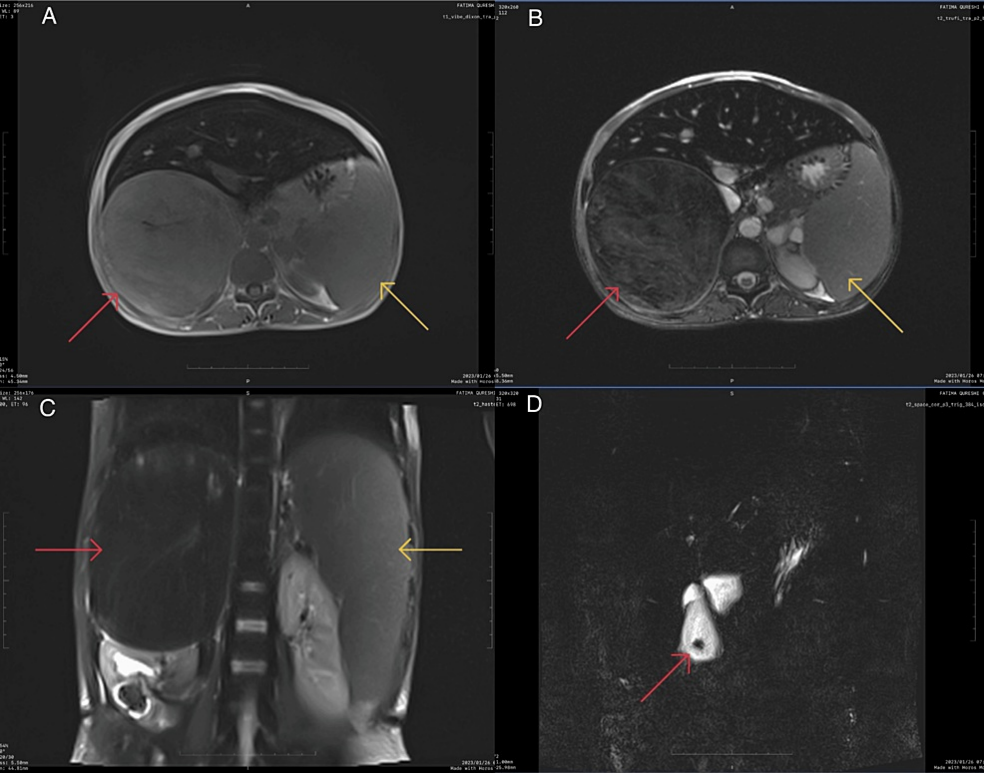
MRCP of the patient The images show (A) T1-weighted axial section of the right suprarenal mass (red arrow) with splenomegaly (yellow arrow); (B) T2-weighted axial section of the right suprarenal mass (red arrow) with splenomegaly (yellow arrow); (C) T2-weighted coronal section of the right suprarenal mass (red arrow) and splenomegaly (yellow arrow); and (D) T2-weighted coronal section with gall bladder calculus (red arrow) MRCP: magnetic resonance cholangiopancreatography
